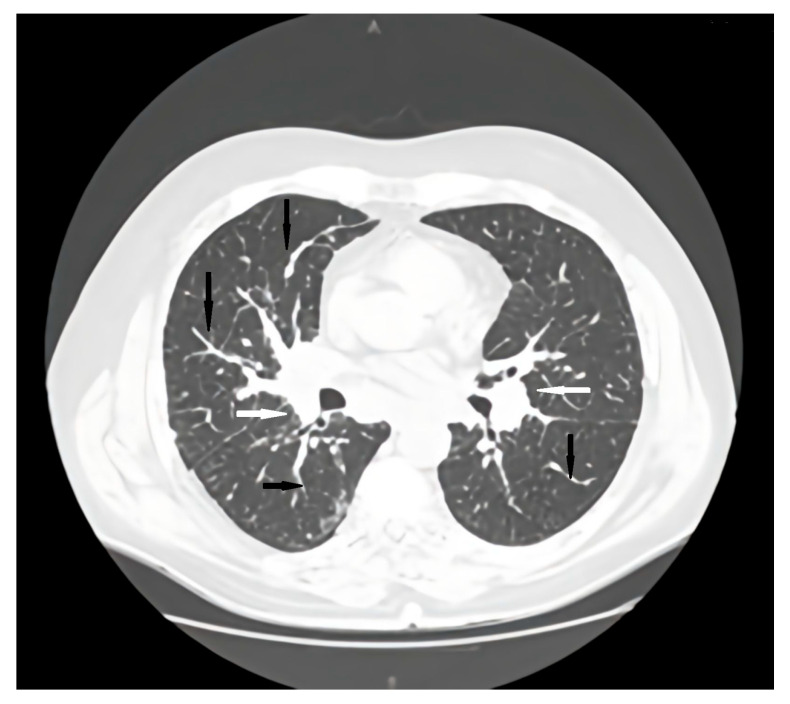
Axial CT scan pulmonary window initial evaluation. A, anterior; P, posterior; black arrows, irregular peribronchovascular thickening; white arrows, adenopathy.
